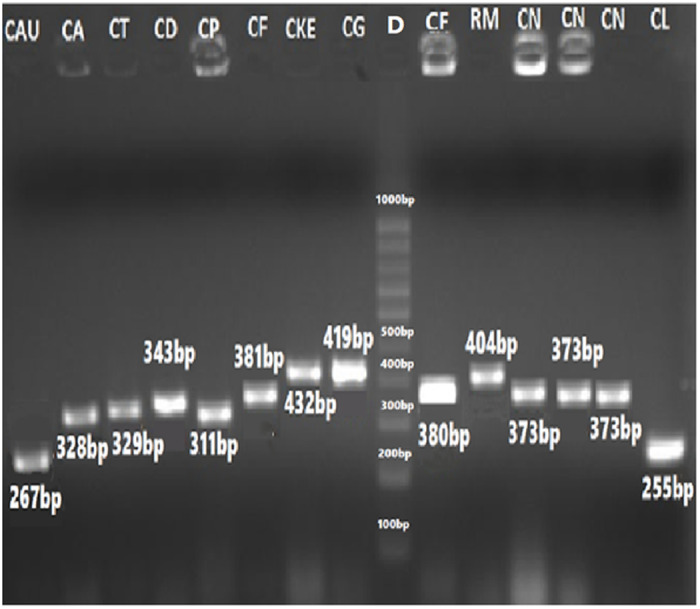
PCR amplified products using ITS3&4 primers of ITS regions of yeast species. CAU= *Candida auris* (267 bp), CA= *C*. *albicans* (328 bp), and CT=*C*. *tropicalis* (329 bp), CD= *C*. *dubliniensis* (343 bp), CP= *C*. *parapsilosis* (311 bp), CF= *C*. *famata* (381 bp), CKE= *C*. *kefyr* (432 bp) CG= *C*. *glabrata* (419 bp), CF= *C*. *famata* (381 bp), RM= *Rhodotorulla mucilaginosa* (404 bp) CN= *Cryptococcus neoformans* (373 bp) CL= *C*. *lusitaniae* (255 bp) as compared to 100 bp DNA ladder (9).
